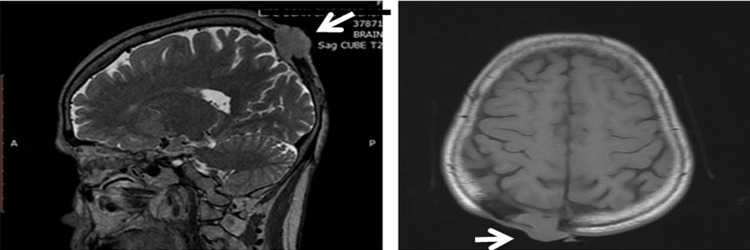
MRI sagittal view An irregular lesion with the destruction of the right parieto-occipital bones involving both inner and outer tables and intracranial extension
